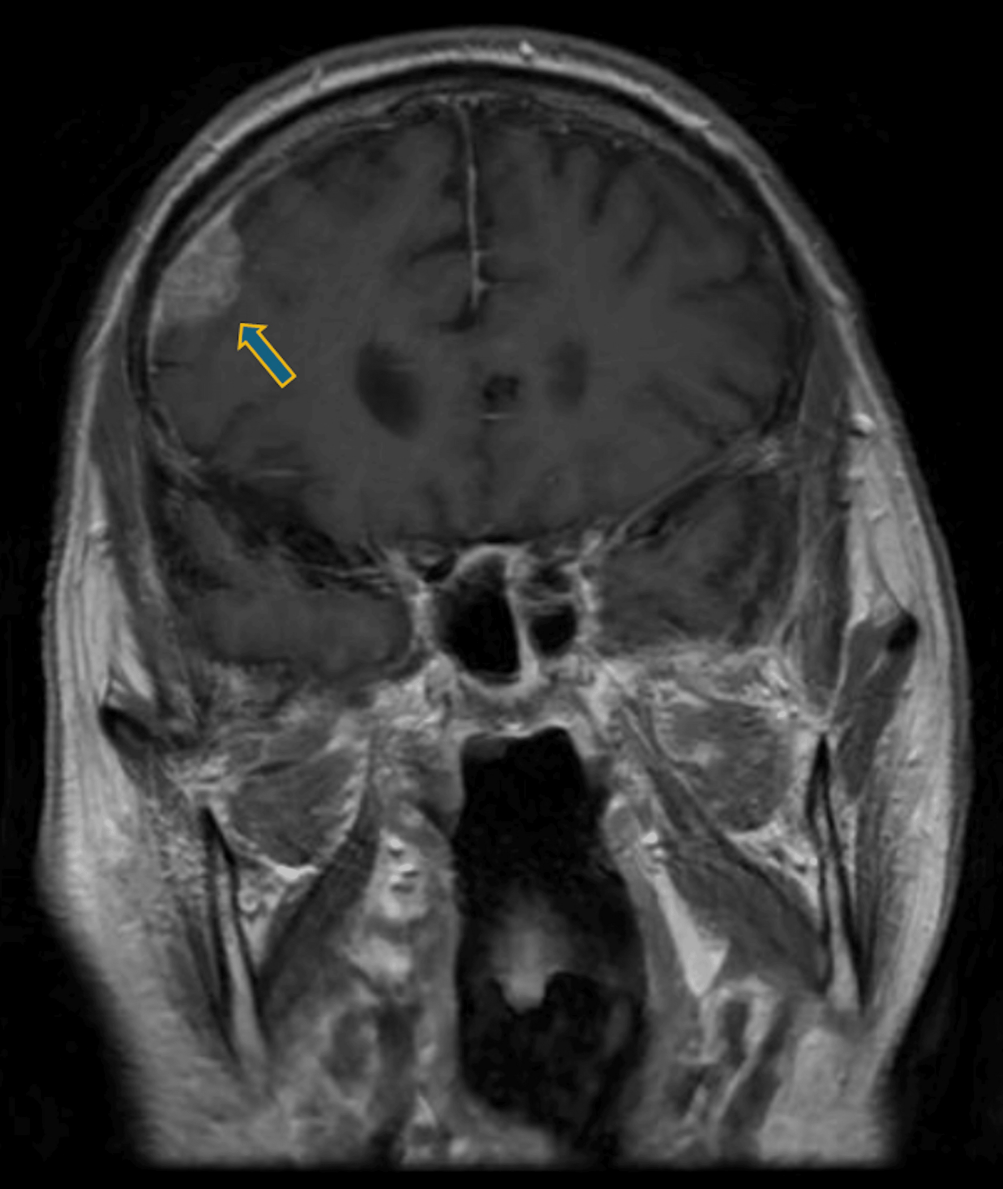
Brain MRI (T1 coronal) with contrast showed a right frontal lobe meningioma.
